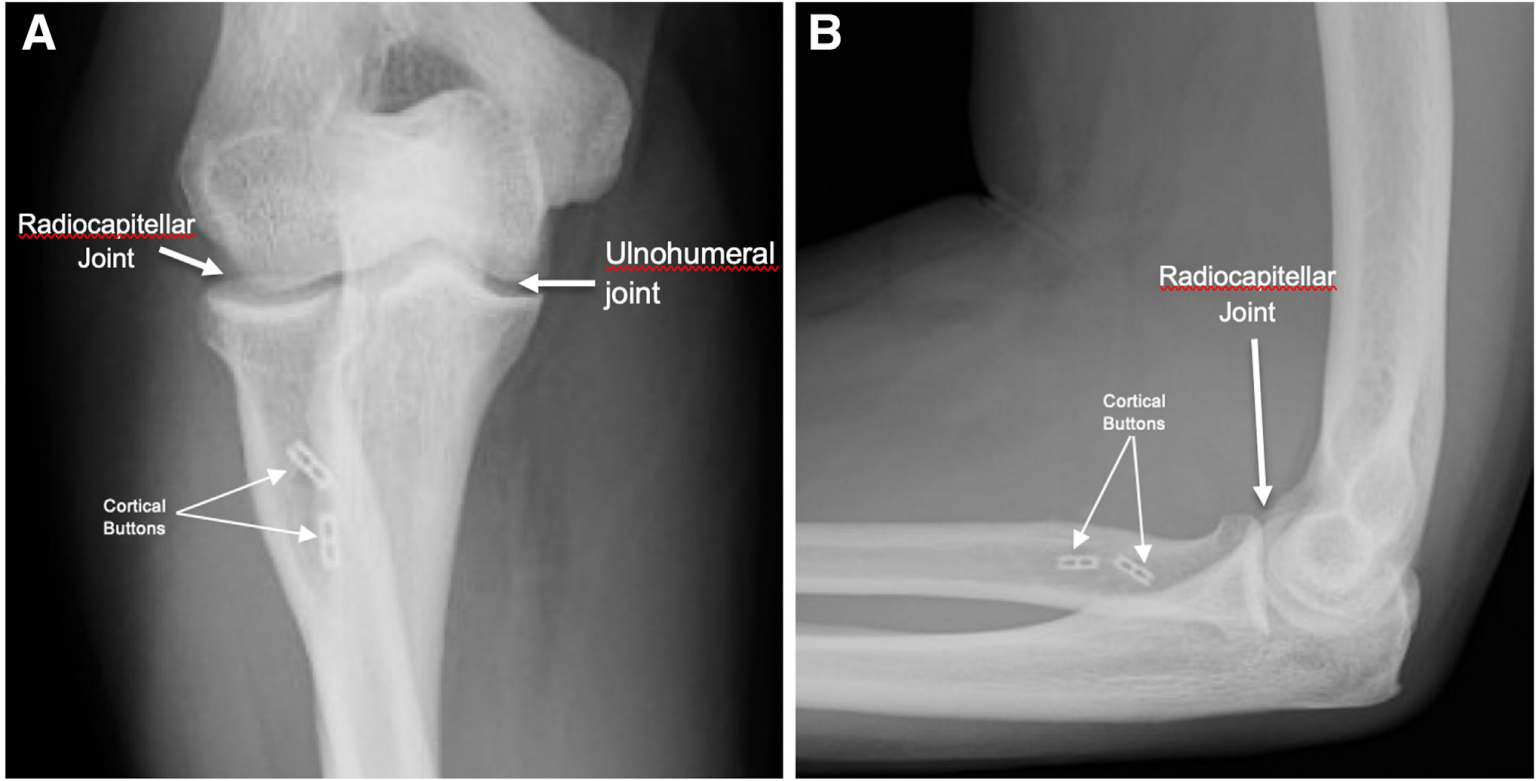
(A) AP radiograph of the elbow after tensioning of intramedullary suture buttons at the radial tuberosity. (B) Lateral radiograph of the elbow showing intact intramedullary suture buttons at the level of the radio tuberosity following final tightening.
